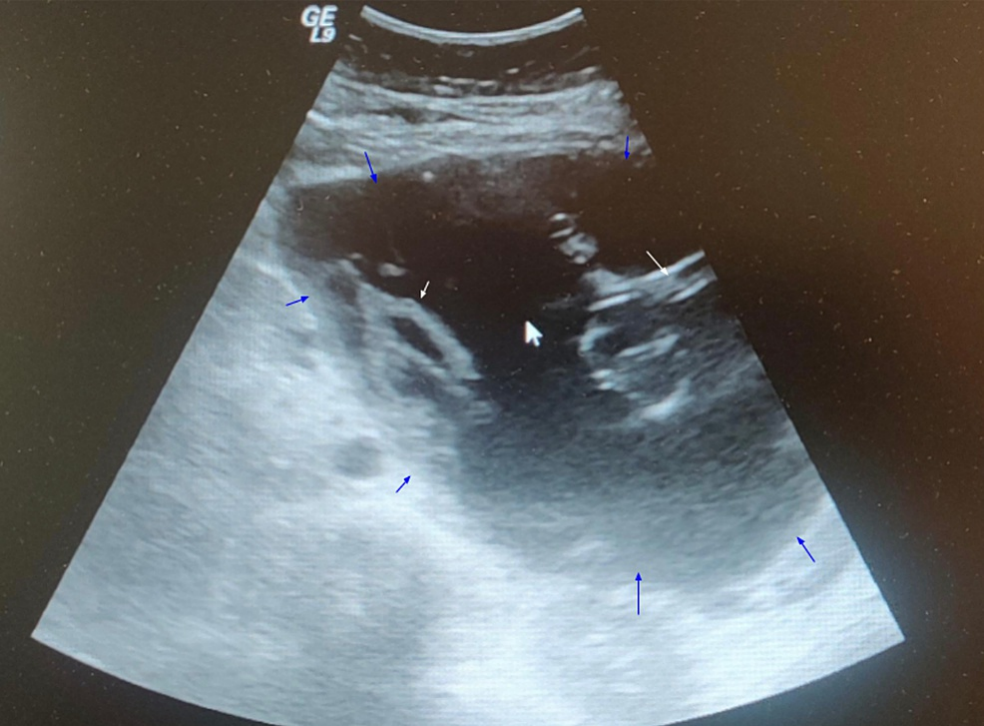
Ultrasound of the pancreatic cyst prior to percutaneous drainage. Blue arrows indicate the boundaries of the pancreatic cyst, and white arrows indicate the septations within the pancreatic cyst.
